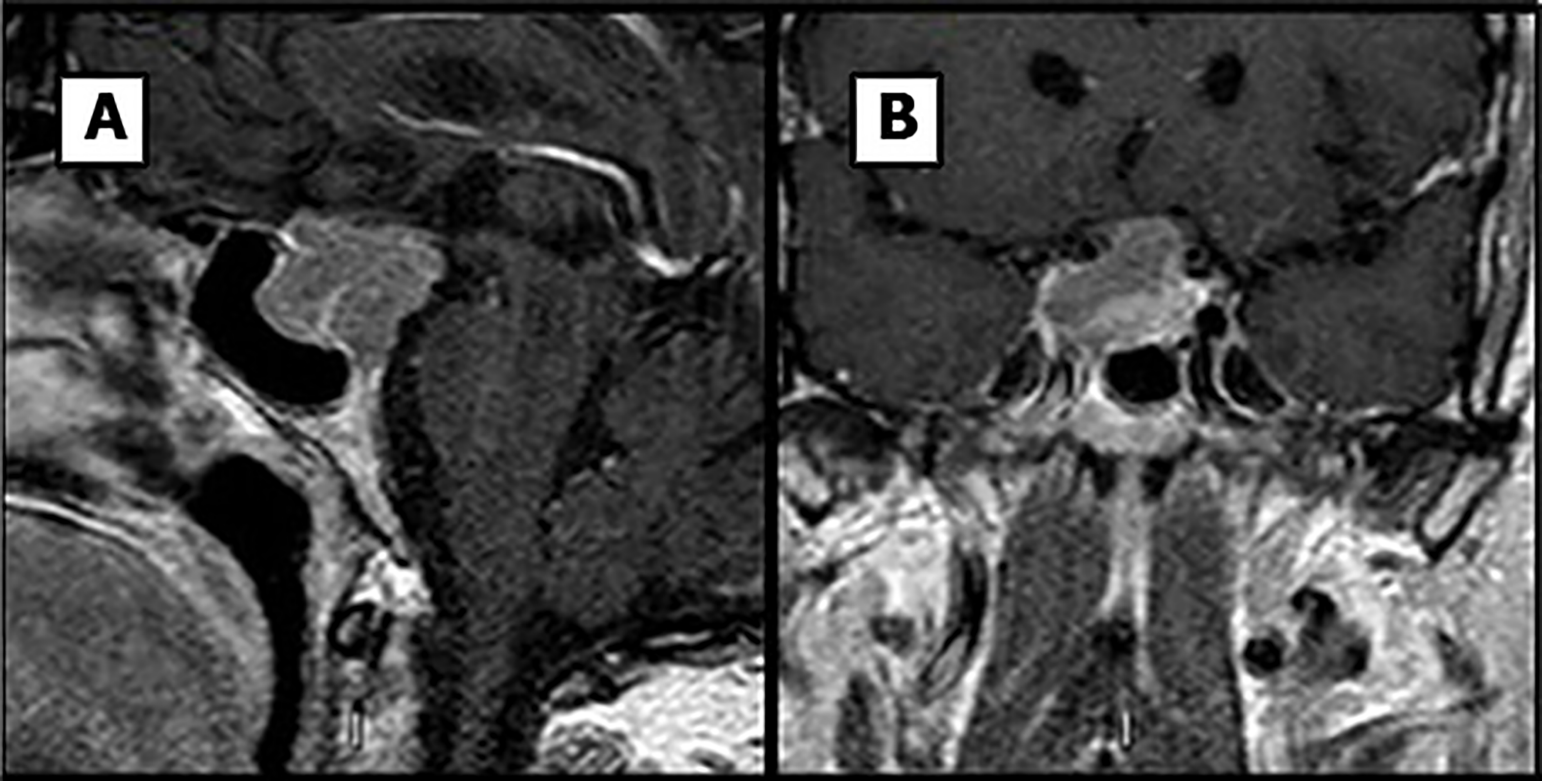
MR images previous to first surgery. Sagital (A) and coronal (B) T1-weighted images.
